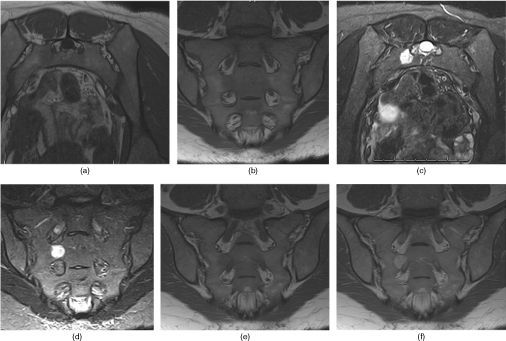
MRI of the pelvis reveals sacral lesion. Axial (a) and coronal (b) *T*_1_ weighted images of the pelvis (scan parameters: TR= 540 ms; TE= 10 ms; 640 × 640; 4-mm slice thickness) demonstrate a slightly hypointense well-demarcated rounded lesion involving the right sacrum at S2 between the anterior neural foramina of S1 and S2. This lesion was hyperintense on axial (c) and coronal (d) short tau inversion recovery (scan parameters: TR = 5400 ms; TE = 60 ms; 512 × 512; 4-mm slice thickness). Coronal pre-contrast (e) and post-contrast *T*_1_ weighted (scan parameters: TR = 700 ms; TE=10 ms; 640 × 640; 4-mm slice thickness) images (f) demonstrate homogeneous enhancement within the right sacral lesion. TE, echo time; TR, repetition time.
